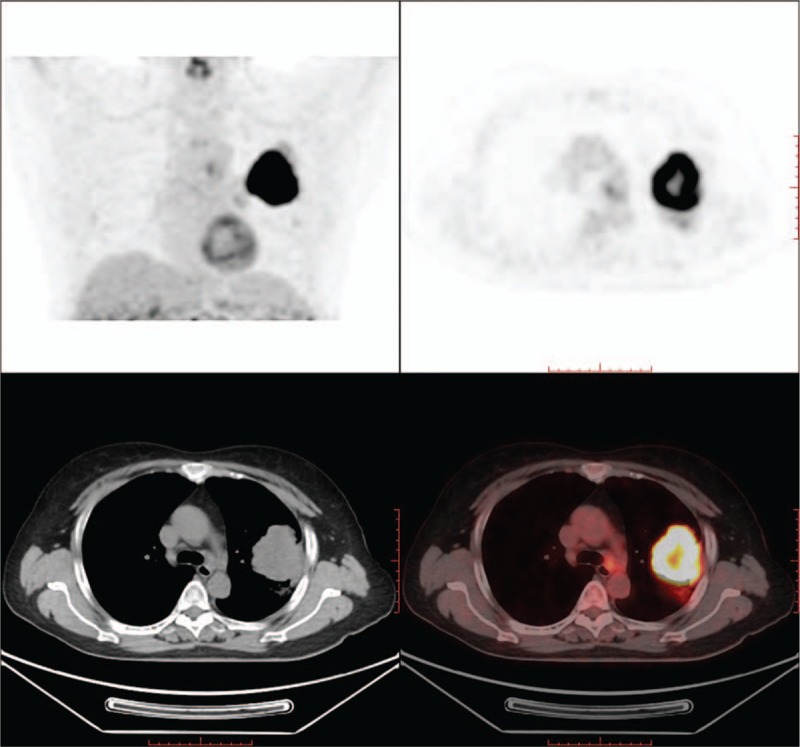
Nonsmoker lung cancer patients positron emission tomography/integrated computed tomography figure. Mediastinal lymph nodes SUVmax value was 2.2 and tumor SUVmax value was 23.9. This positron emission tomography/integrated computed tomography positive mediastinal lymph nodes turns out to be pathological benign.
